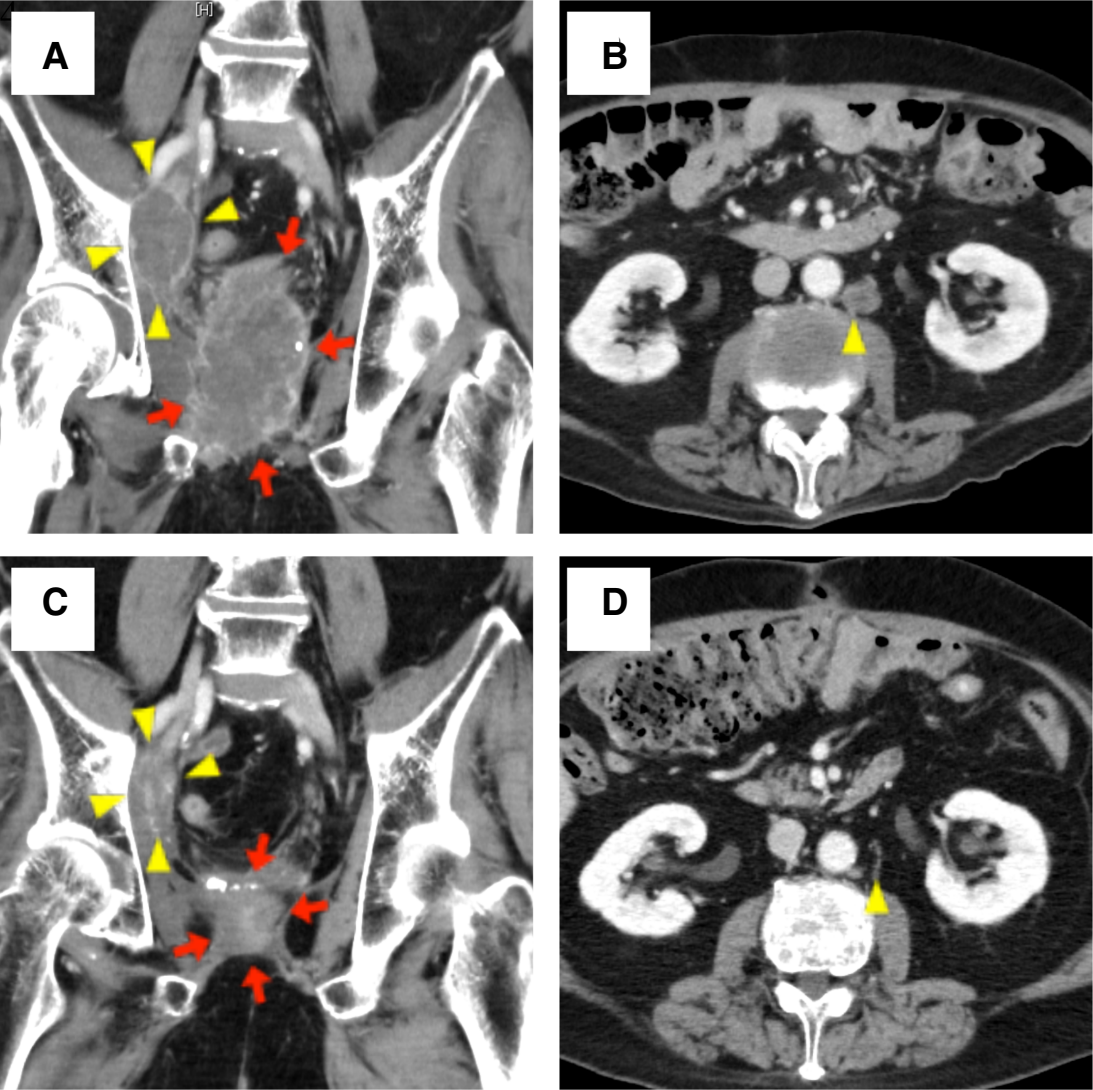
Images of abdominal contrast–enhanced computed tomography before (*A*,*B*), and 10 mo after (*C*,*D*) the start of pembrolizumab treatment. (*A*,*C*) Coronal images show a remarkable reduction in the size of the local lesion and the right obturator lymph node. (*B*,*D*) Axial images showing the disappearance of the para-aortic lymph node.
